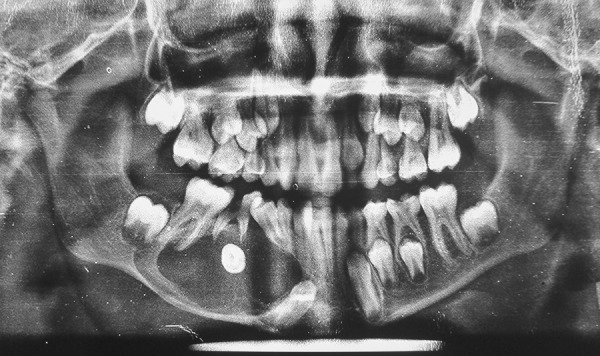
Preoperative panoramic radiograph showing well-defined periapical unilocular radiolucency
